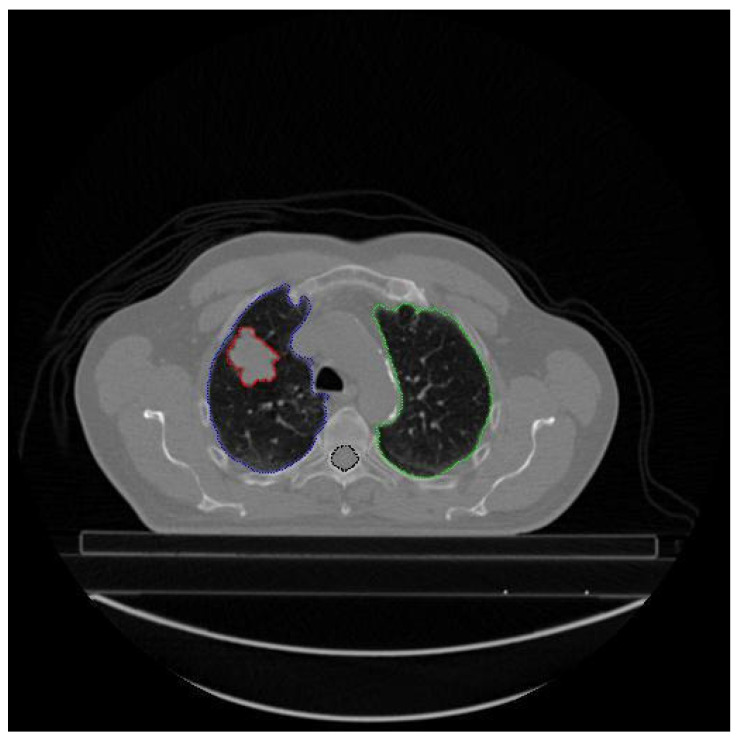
Different types of segmentation data contained in a CT slice (28) of a patient (LUNG1-243).
